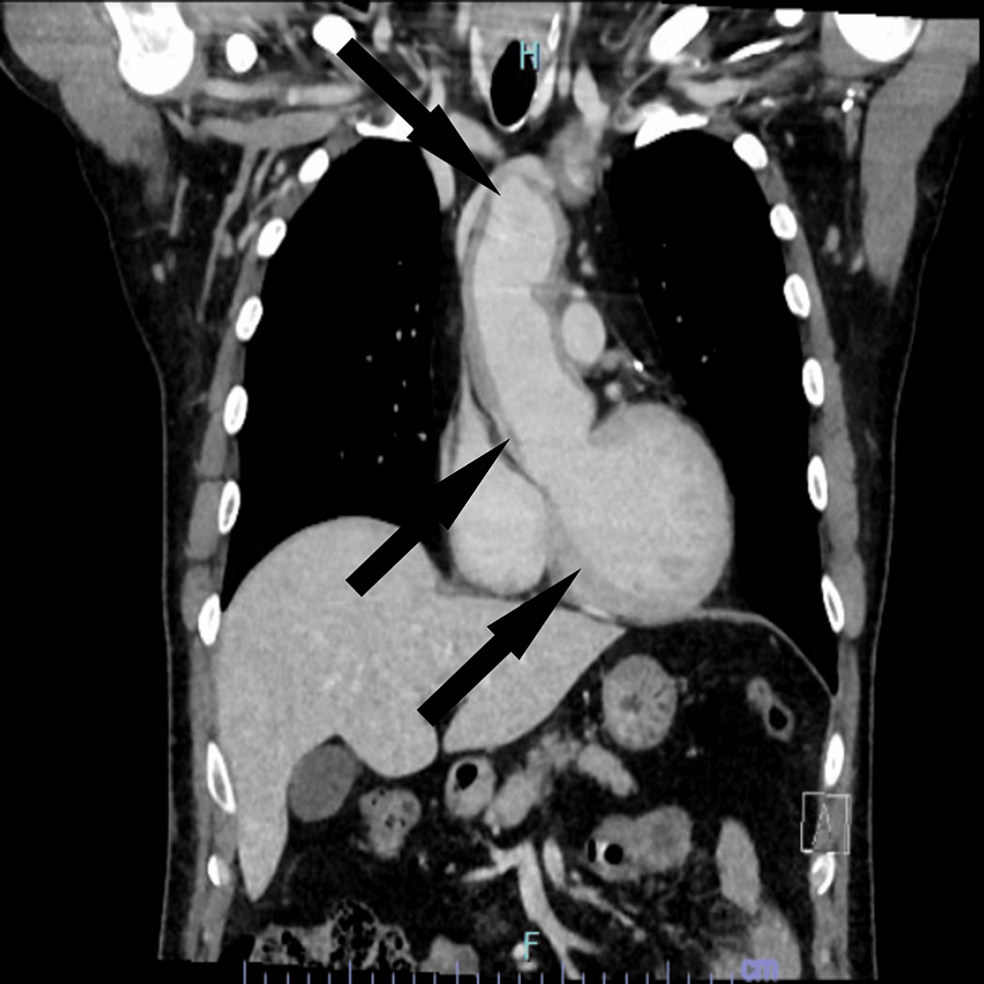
Computed tomography angiography of the chest showing thickening of the walls of the aorta and aneurysmal dilation
